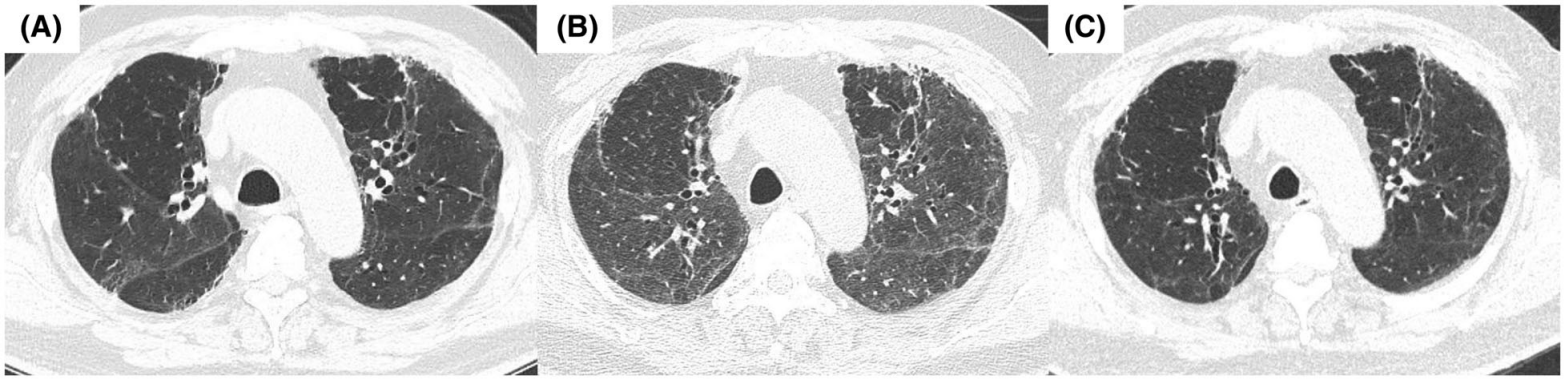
High‐resolution computed tomography (CT) images before vaccination (A: December 2020), 1 day after COVID‐19 mRNA vaccination (B: July 2021) and after treatment (C: September 2021). After vaccination, diffuse ground‐glass opacities (GGOs) were superimposed on pre‐existing reticular opacities. The GGOs on chest CT were ameliorated after the treatment
